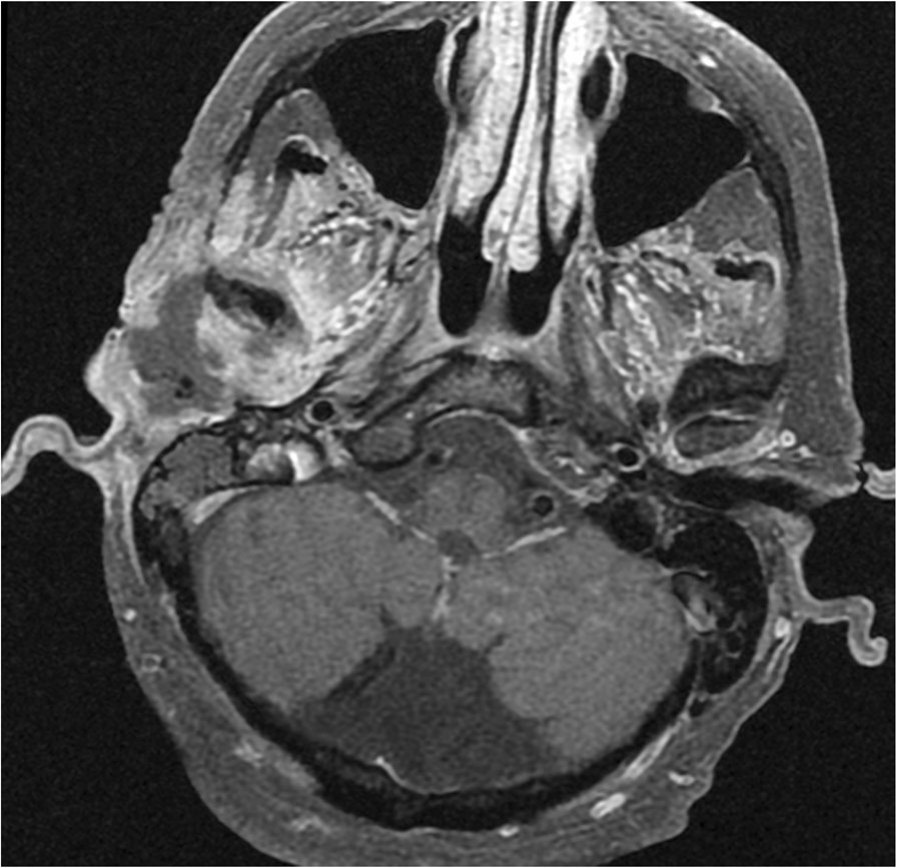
External auditory canal squamous cell carcinoma. Axial fat-suppressed post-contrast T1-weighted MRI shows an infiltrative and necrotic mass centered in the right external auditory canal with extension into the right temporomandibular joint space
